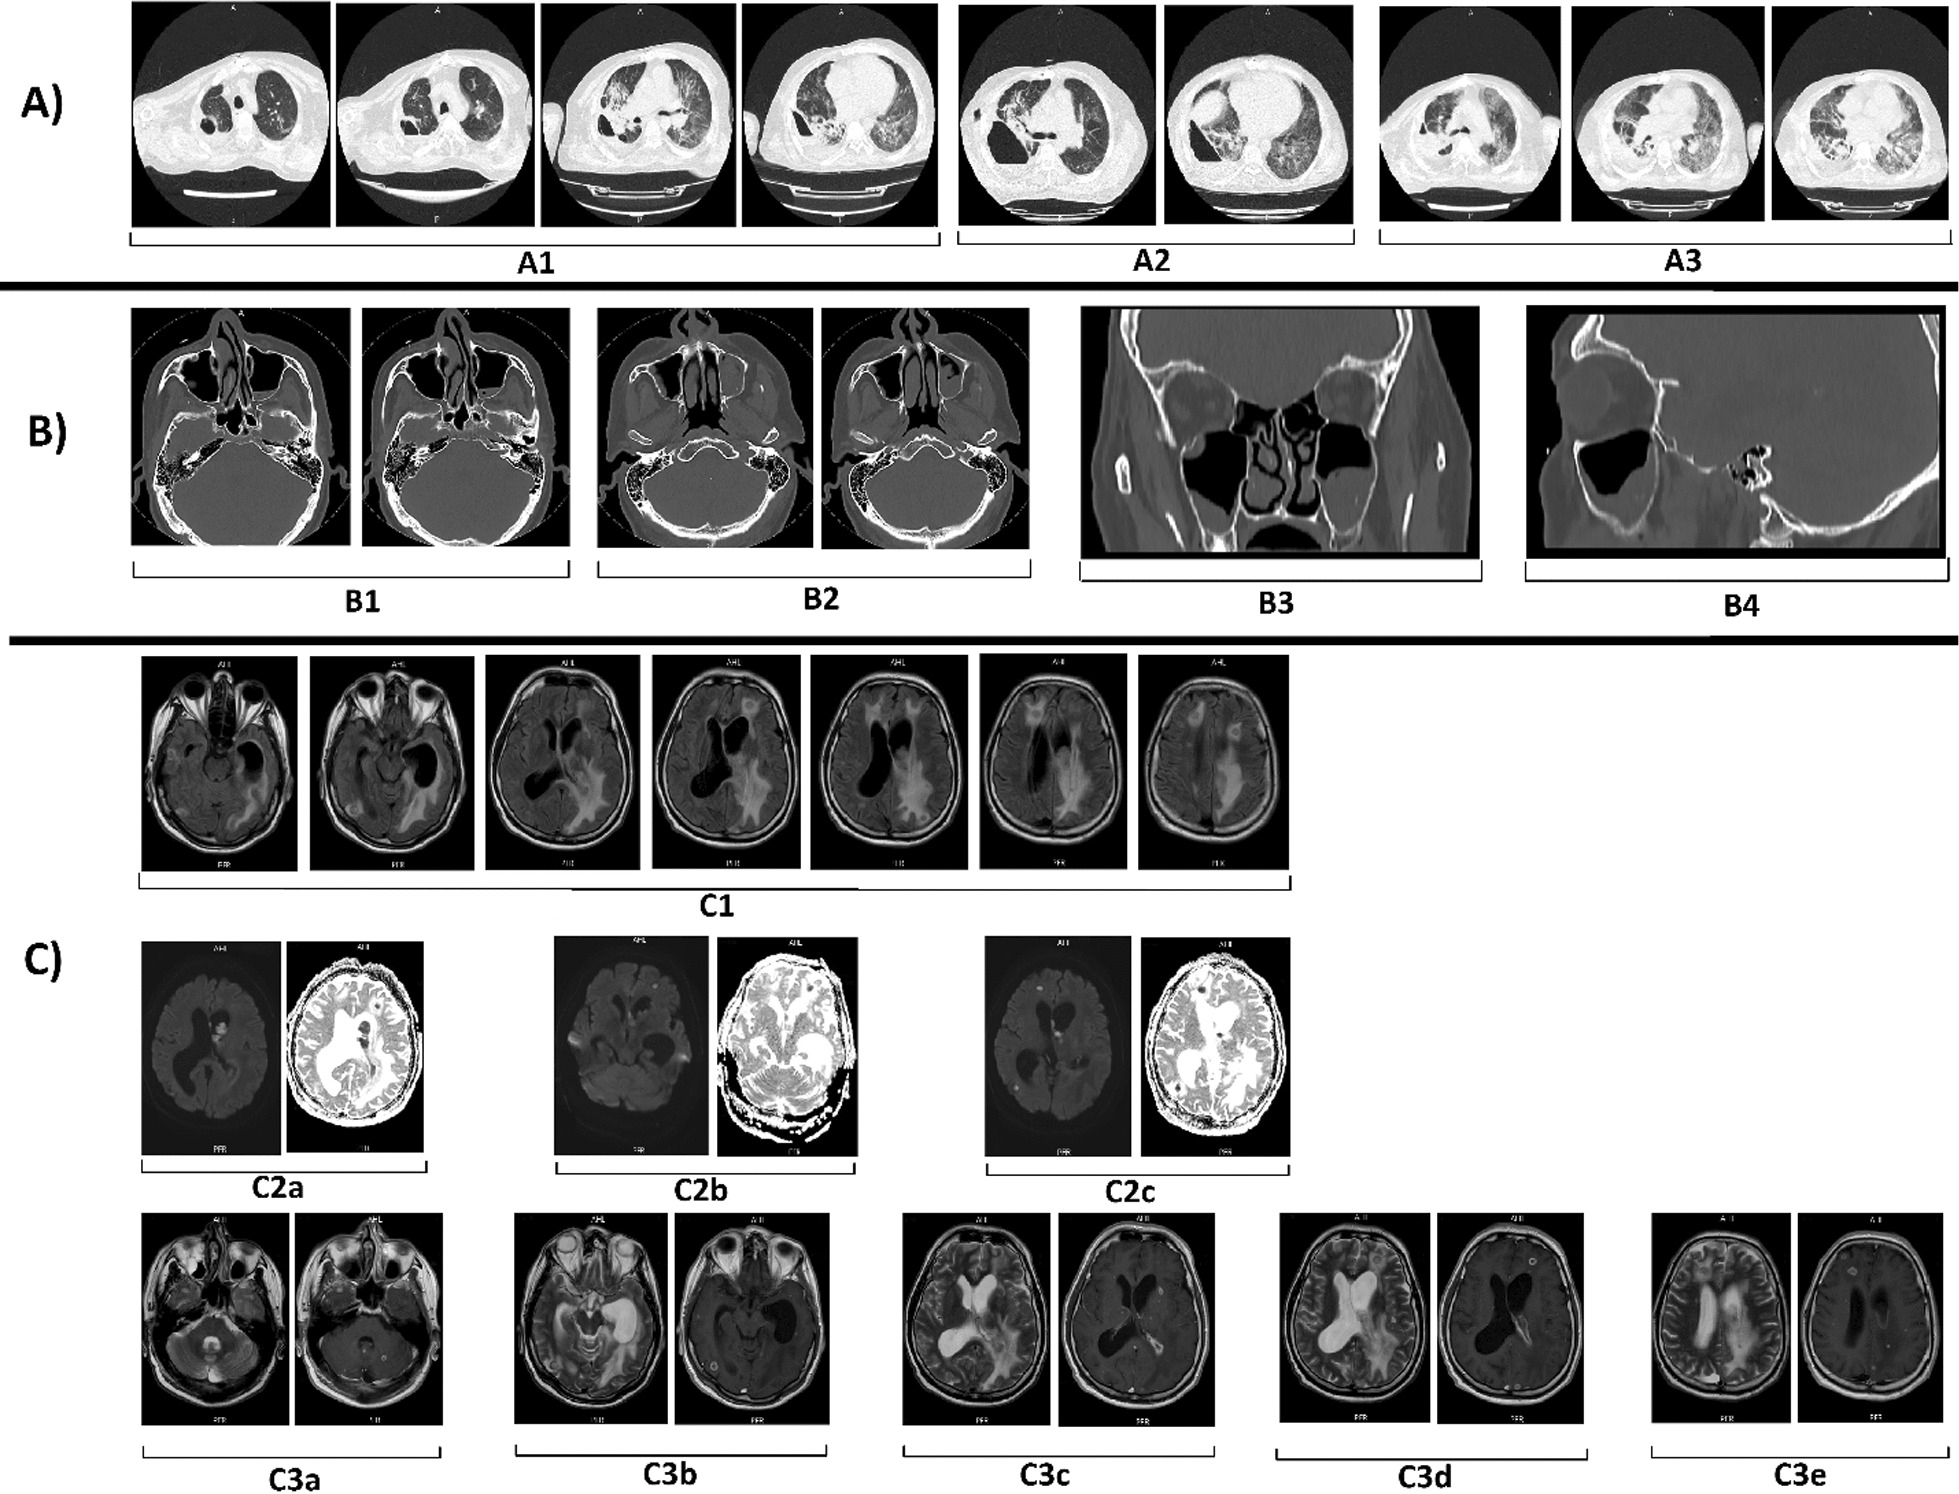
A Computerized tomography (CT) scan of the lungs at admission day (A1), 11 days (A2), and 26 days (A3) later. There is a right-sided loculated hydropneumothorax connected to a parenchymal abscess. Lung parenchyma shows widespread ground glass with some reticular opacities bilaterally. B Paranasal sinus CT scan on day 5 of admission shows mucosal thickening in maxillary sinuses, air bubbles, and air-fluid level in left maxillary sinuses in favor of acute sinusitis (B1). Punctuate, linear, and irregular calcifications in the axial (B2 left plane), coronal (B3), and sagittal (B4) views and a round nonfluid collection (B2 right plane) are visible in the left maxillary sinus, together suggesting an underlying fungus ball with superimposed acute sinusitis. C Brain MRI on day 2 of admission. In the upper row (C1) in the fluid-attenuated inversion recovery sequences of MRI (FLAIR) there are multiple high-signal round mass-like lesions throughout the brain parenchyma with cortico-subcortical junction distribution and with significant peripheral vasogenic edema. There is high signal material in the left lateral ventricle and asymmetrical dilation of the ventricles with intraventricular septal deviation to right (noncommunicating hydrocephalus). In the middle row, diffusion-weighted imaging (DWI) (C2a–c left planes) and apparent diffusion coefficient (ADC) (C2a–c right planes) sequences, almost all the mass-like lesions as well as the intraventricular material have shown diffusion restriction. In the bottom row, T2 (C3a–e left planes) and contrast-enhanced T1 MRI sequences (C3a–e right planes), the mass-like lesions have ring enhancement, and the intraventricular collection has shown thick enhancement in close relationship to the ependymal lining of the left lateral ventricle. All the above findings are in favor of multiple brain abscesses and ventriculitis with significant vasogenic brain edema and noncommunicating hydrocephalus
